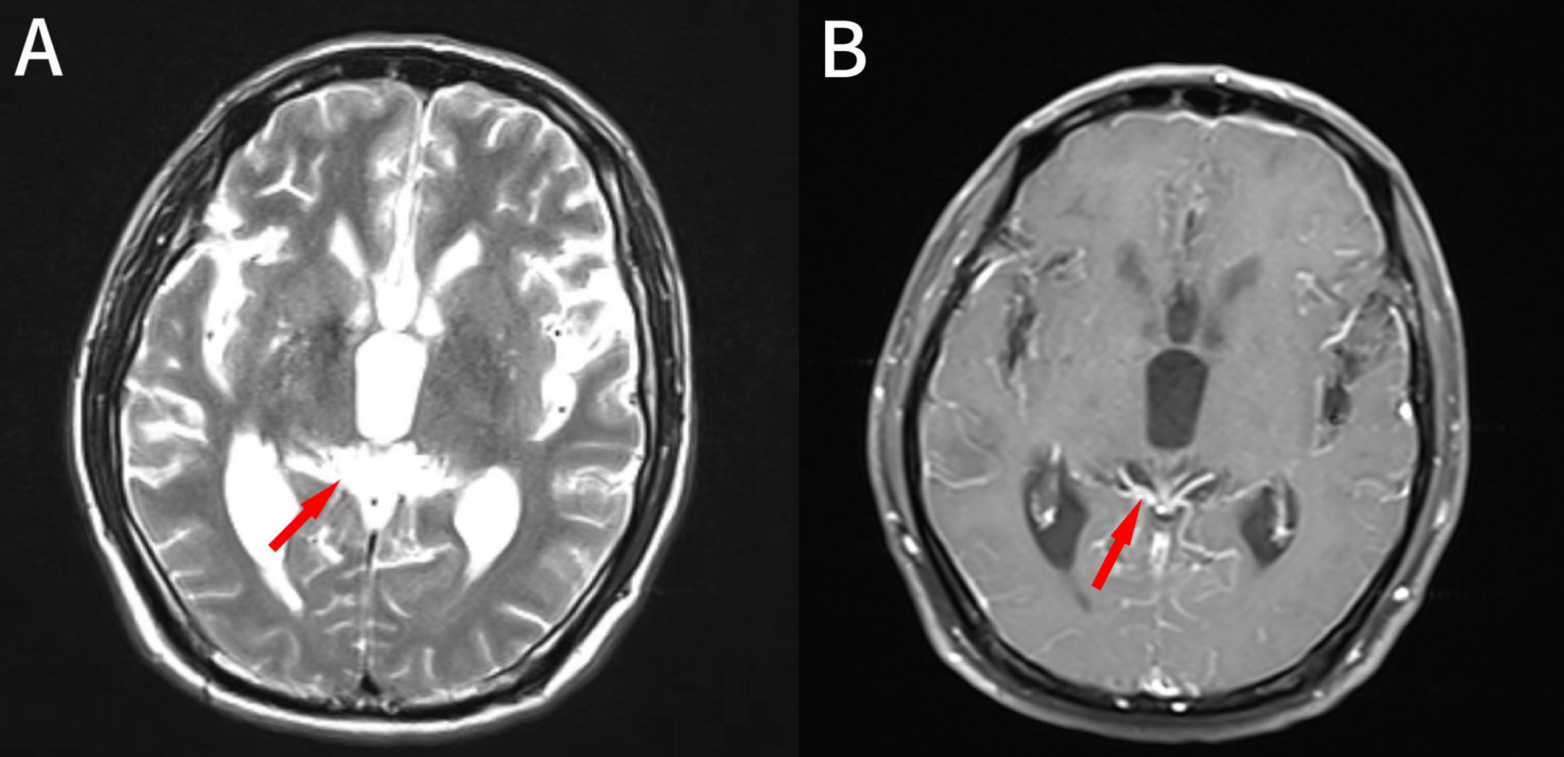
(A, B) The patient’s cranial MRI shows meningeal enhancement.
